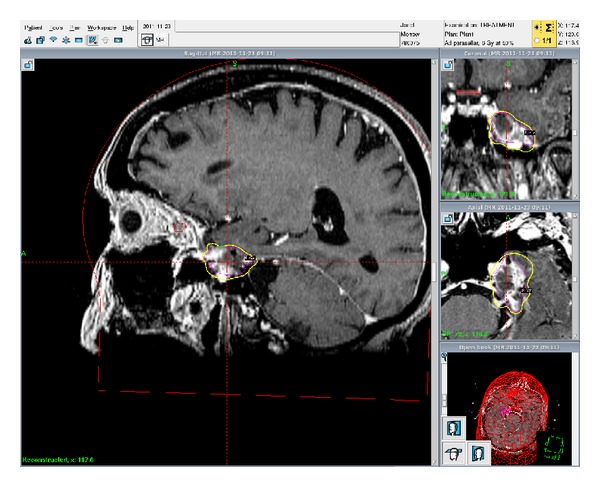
Sagittal treatment planning MRI for the patient's second GKRS treatment showing increased size and the tumor with the Gamma Knife isodose lines in coronal, sagittal, and axial views.
